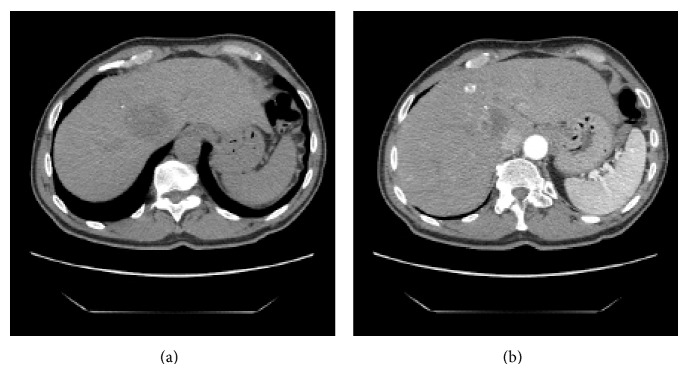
Pretreatment CT scan (a) CT image showing the main tumor in the right lobe of the liver at the confluence of the right and middle hepatic veins measuring 42 × 35 mm. (b) CT-image in the arterial phase showed the second lesion in segment 4 measuring 12 mm in diameter.
